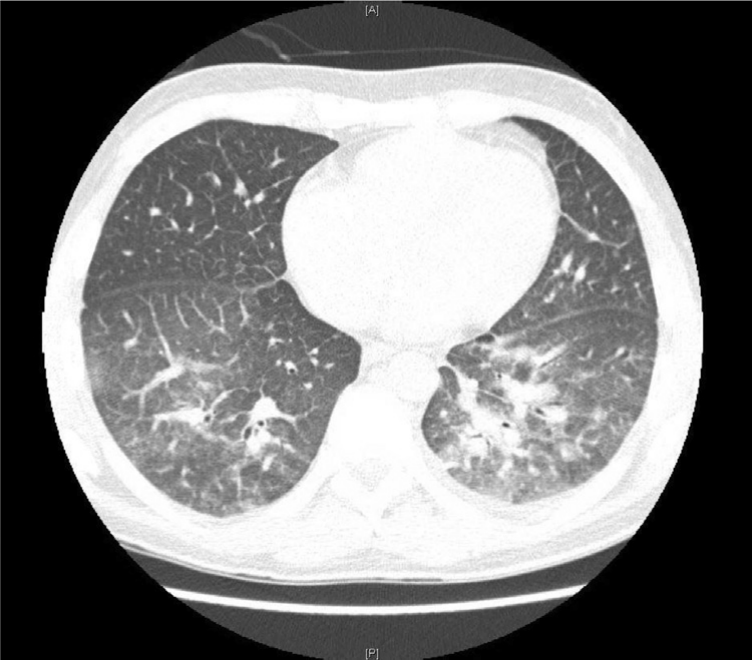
CT chest ordered for hypoxemia and showed ground-glass opacities in the lung bases.
